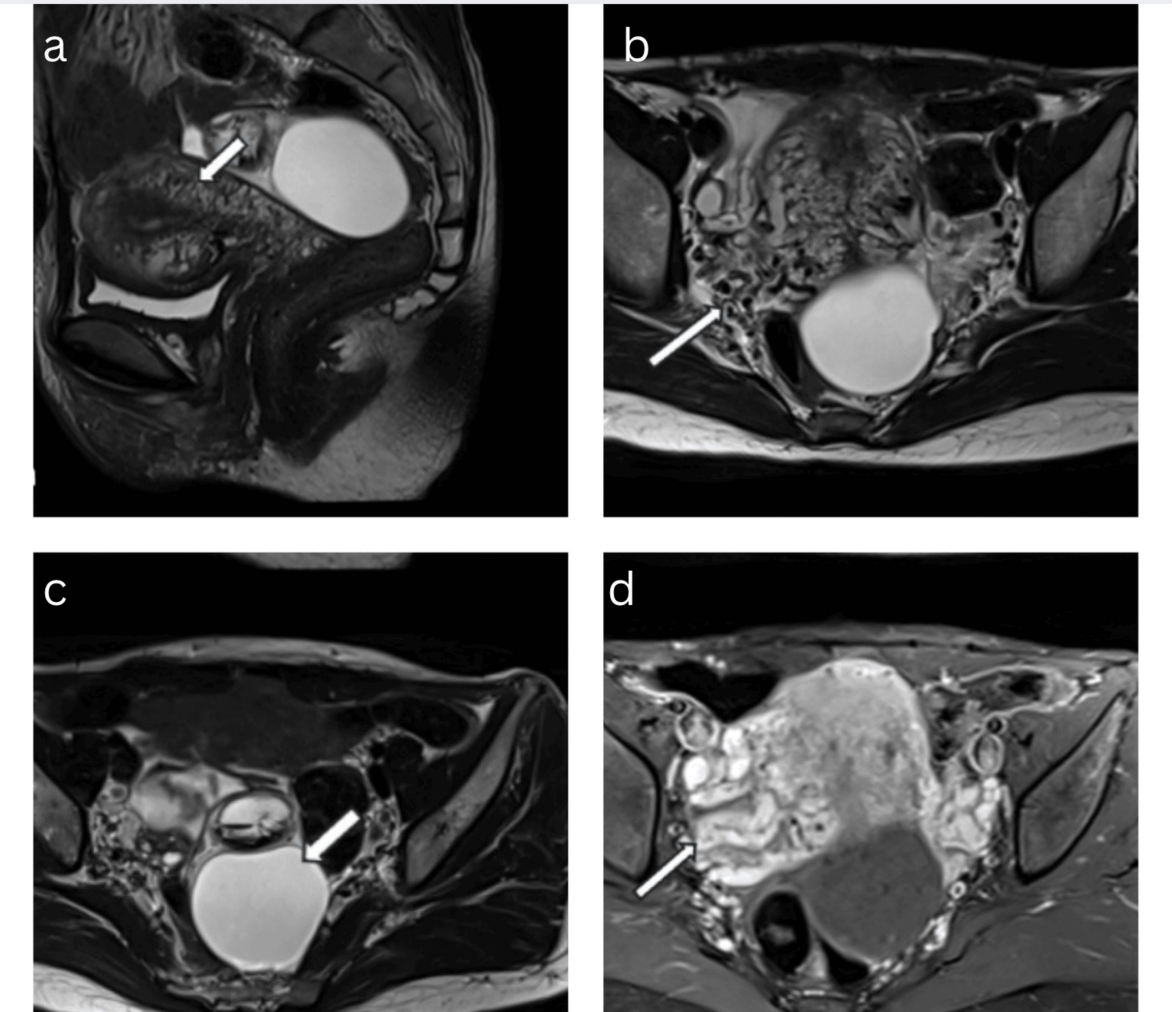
Contrast-enhanced MRI of the pelvis. a, b: Sagittal and axial T2-weighted image showing a bulky uterus with serpiginous vessels in the myometrium and parametrium. c: Axial T2-weighted image showing a simple ovarian cyst and a hemorrhagic cyst in the left ovary. d: Axial post-contrast images showing multiple dilated, tortuous vessels in the myometrium and parametrium.
